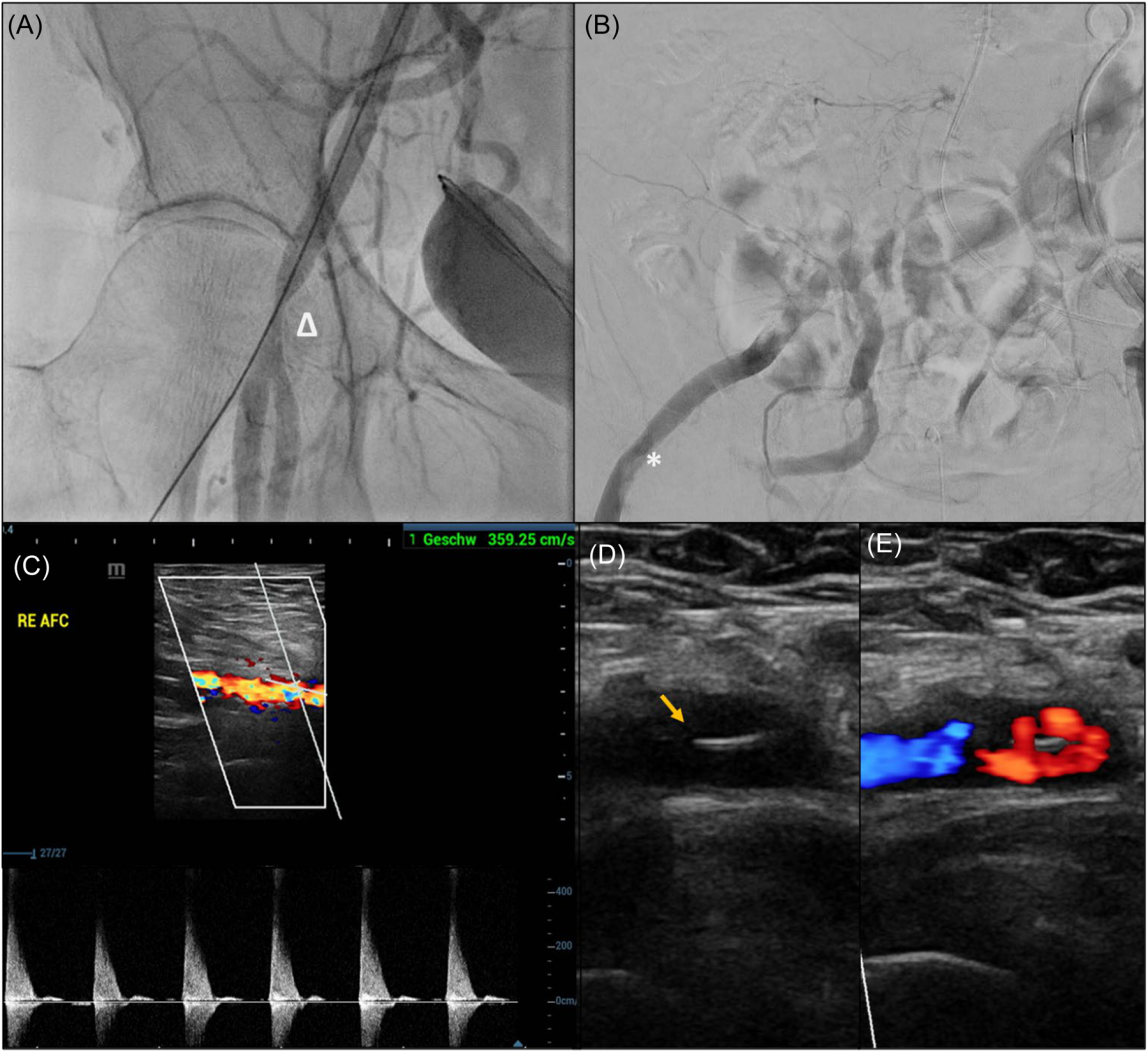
Imaging of femoral arteries after transcatheter aortic valve replacement (TAVR) with stenotic flow profile (A) fluoroscopy of femoral artery stenosis after endovascular closure (Δ) (B) fluoroscopic view of a femoral artery dissection after endovascular closure (*) (C) sonography of a stenotic flow profile of the femoral artery (D) sonography of dissection membrane (arrow) (E) color flow profile of femoral artery dissection.
